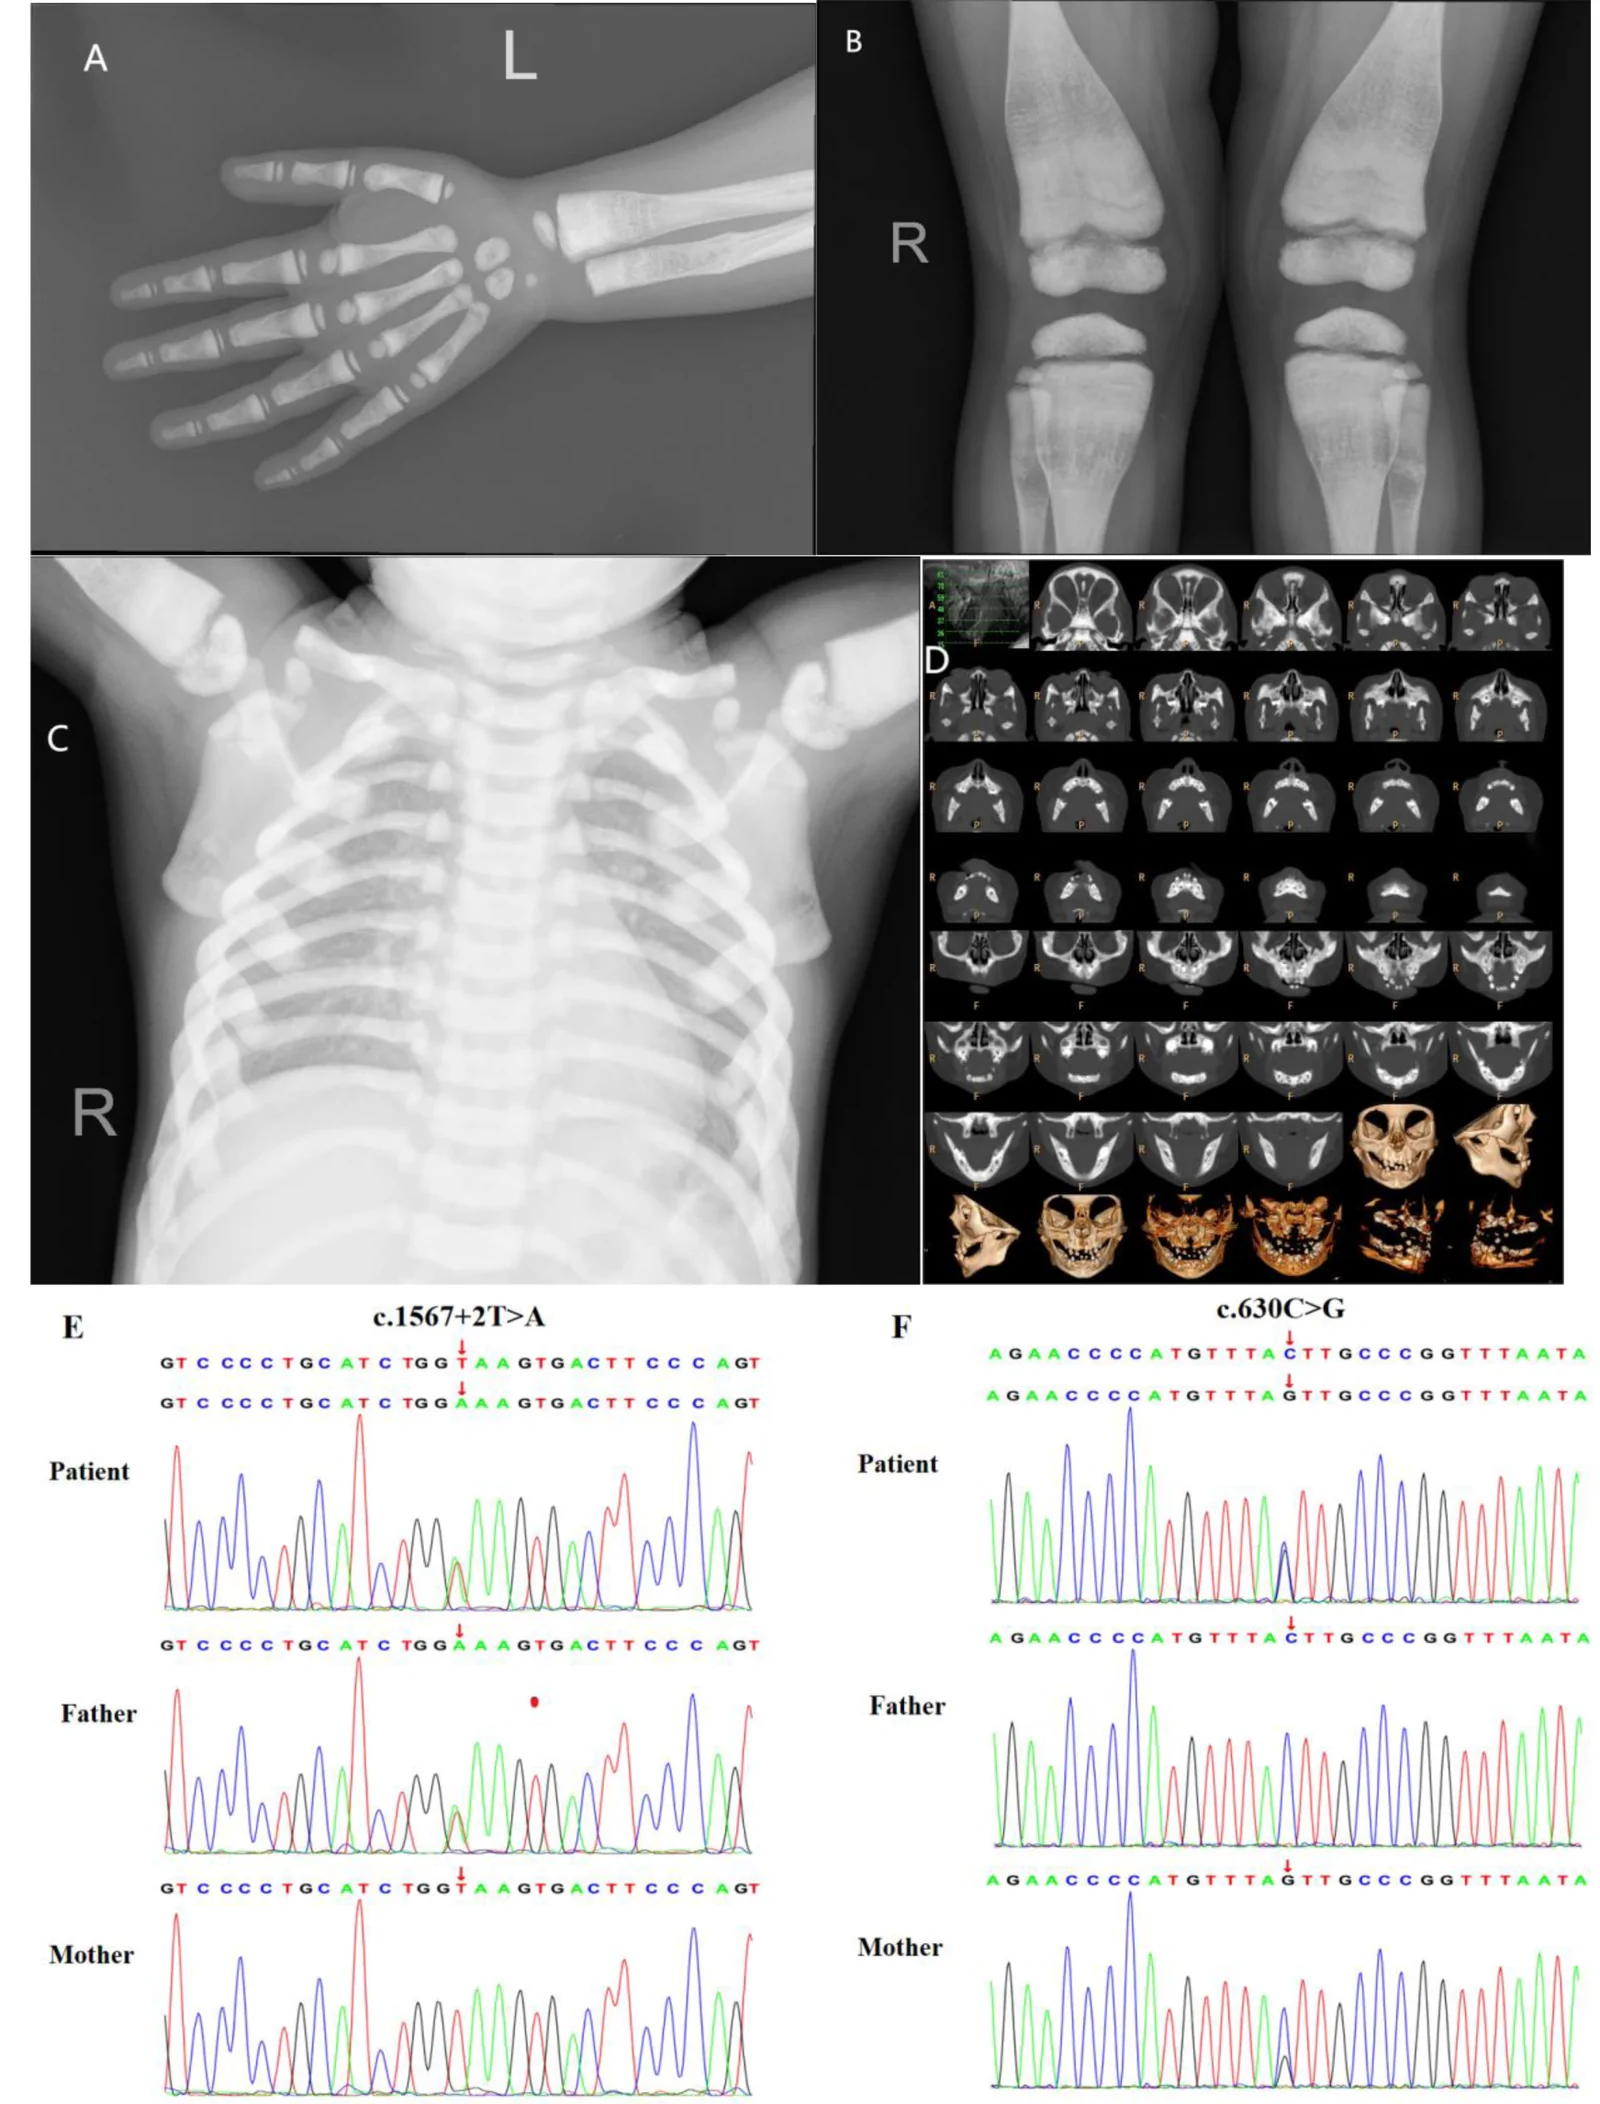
Anteroposterior x-ray of (A) the left wrist; (B) the knees; and (C) the chest. (D) Results of maxillofacial computed tomography + orthopedic 3D imaging. Variation sites of the *TNFRSF11A* gene (red arrows) inherited from the father (E) and the mother (F), detected by whole-exome sequencing.
